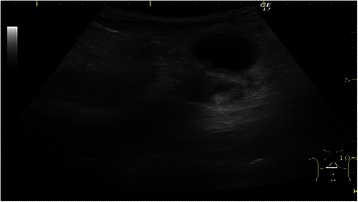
Abdominal ultrasound: pancreatic pseudocyst.
